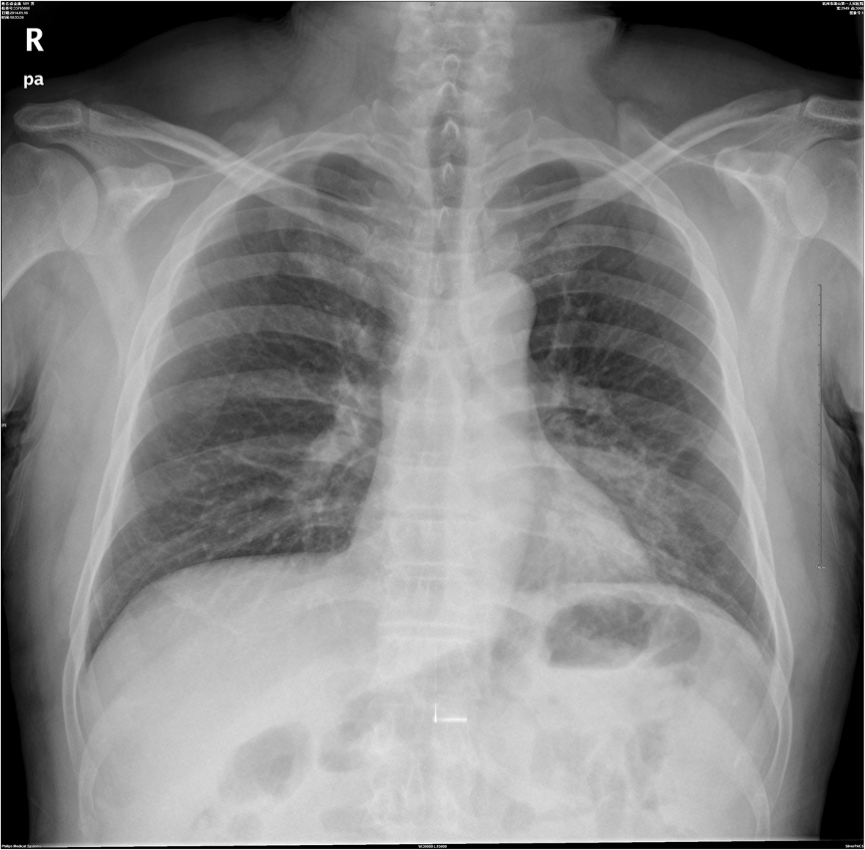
Chest radiograph of the Index case on three days of illness.
